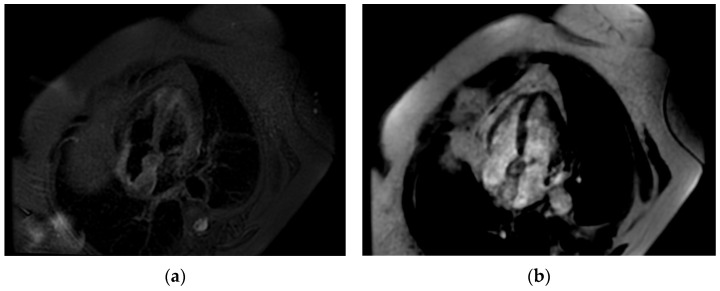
Cardiac MRI. (a) Triple-inversion-recovery T2 sequence of isointense interatrial septum; (b) late gadolinium enhancement (LGE)—enhancement in interatrial septum.
